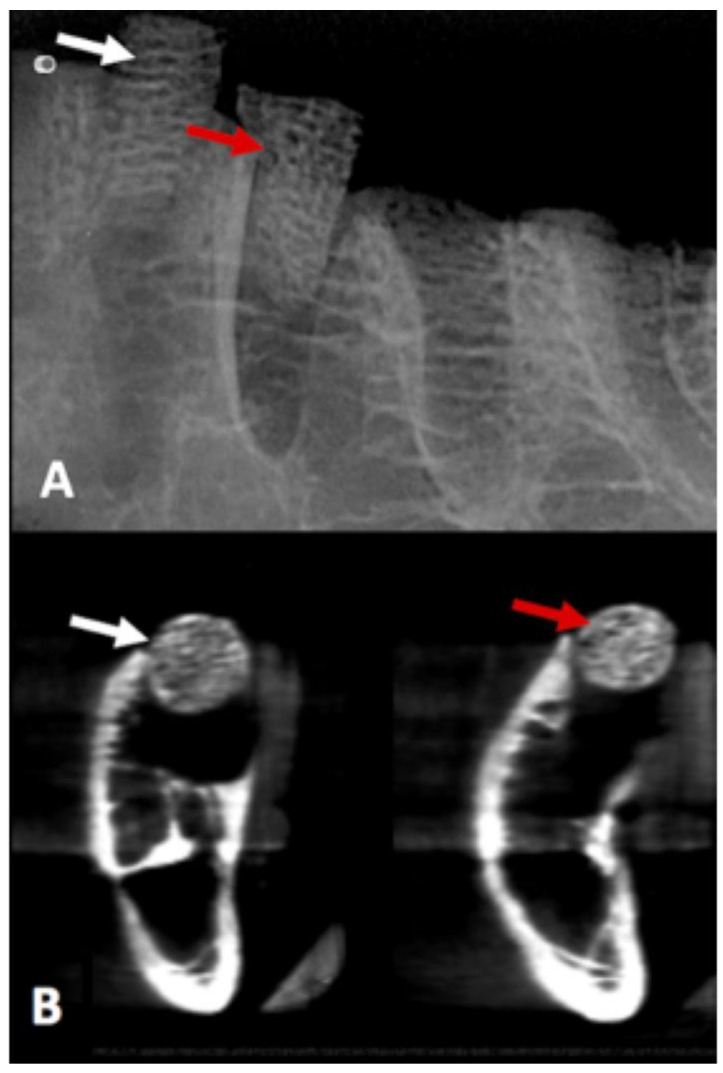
The white arrow shows the bone disc without AgNP impregnation, and the red arrow shows the bone disc with AgNP impregnation at the periapical digital radiograph (A) and CBCT scan (B). The radiographs demonstrated no difference in radio-opacity between the graft impregnated or not with AgNP.
